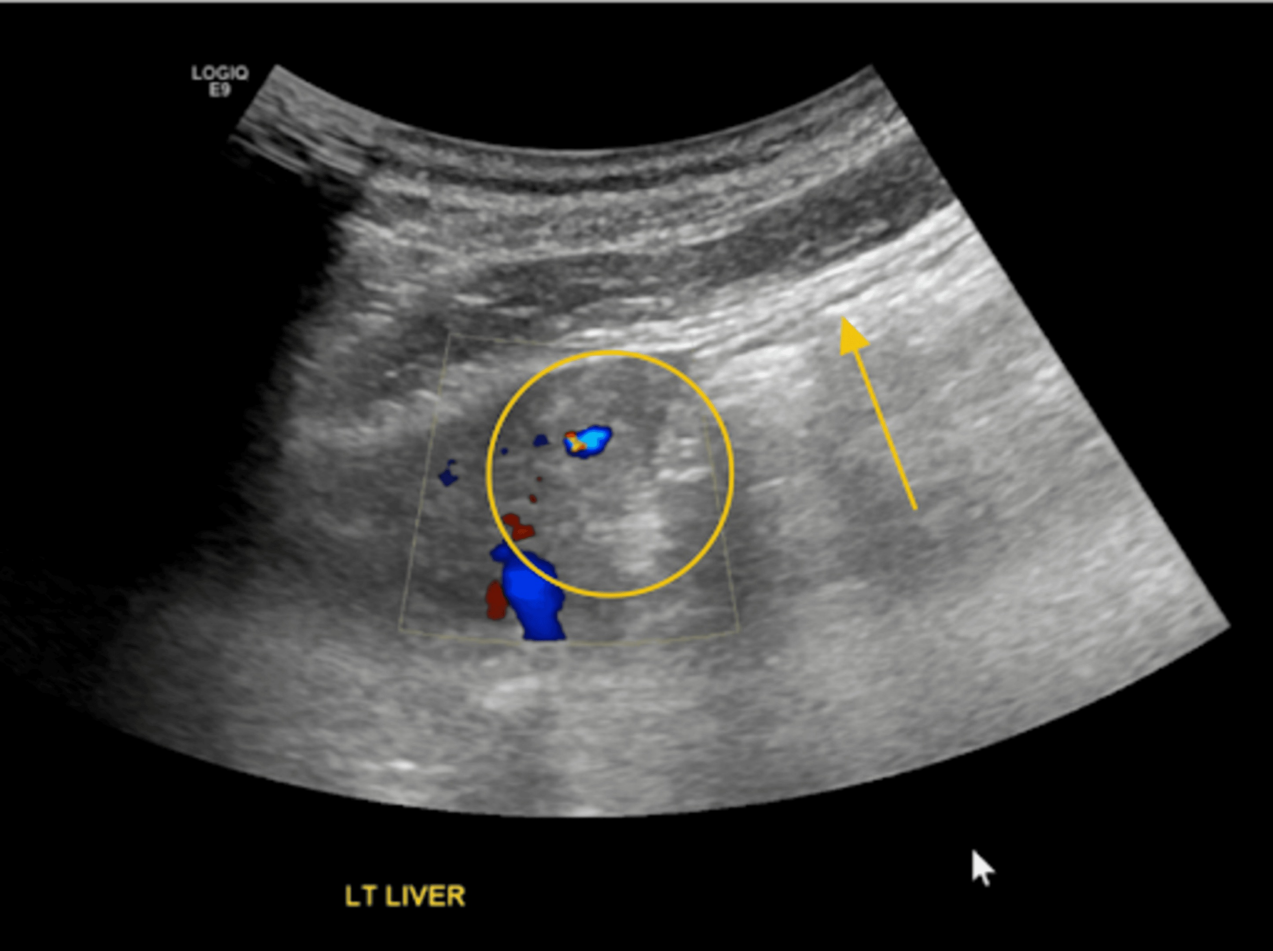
Ultrasound-guided liver biopsy A 19-gauge coaxial needle was advanced to the margin of the left hepatic mass. The needle (yellow arrow) is shown within the hepatic mass (yellow circle).
